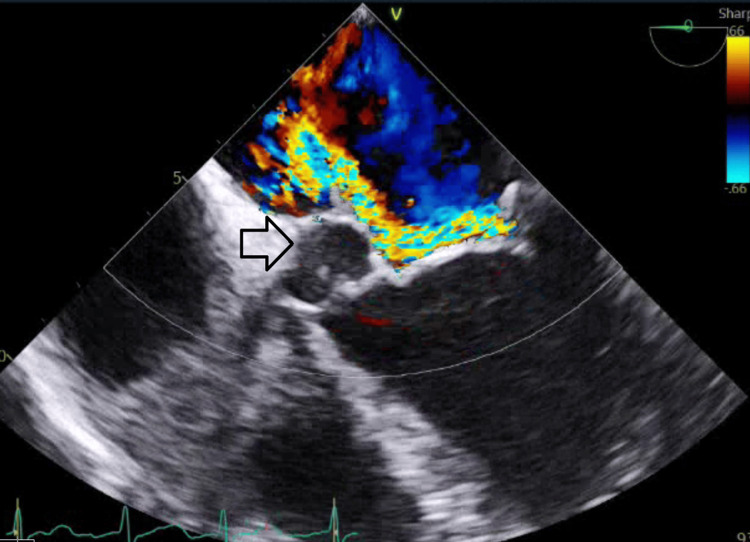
Initial transesophageal echocardiography (TEE) image from hospitalization. The arrow demonstrates the abscess in the mitral valve annulus on TEE.
